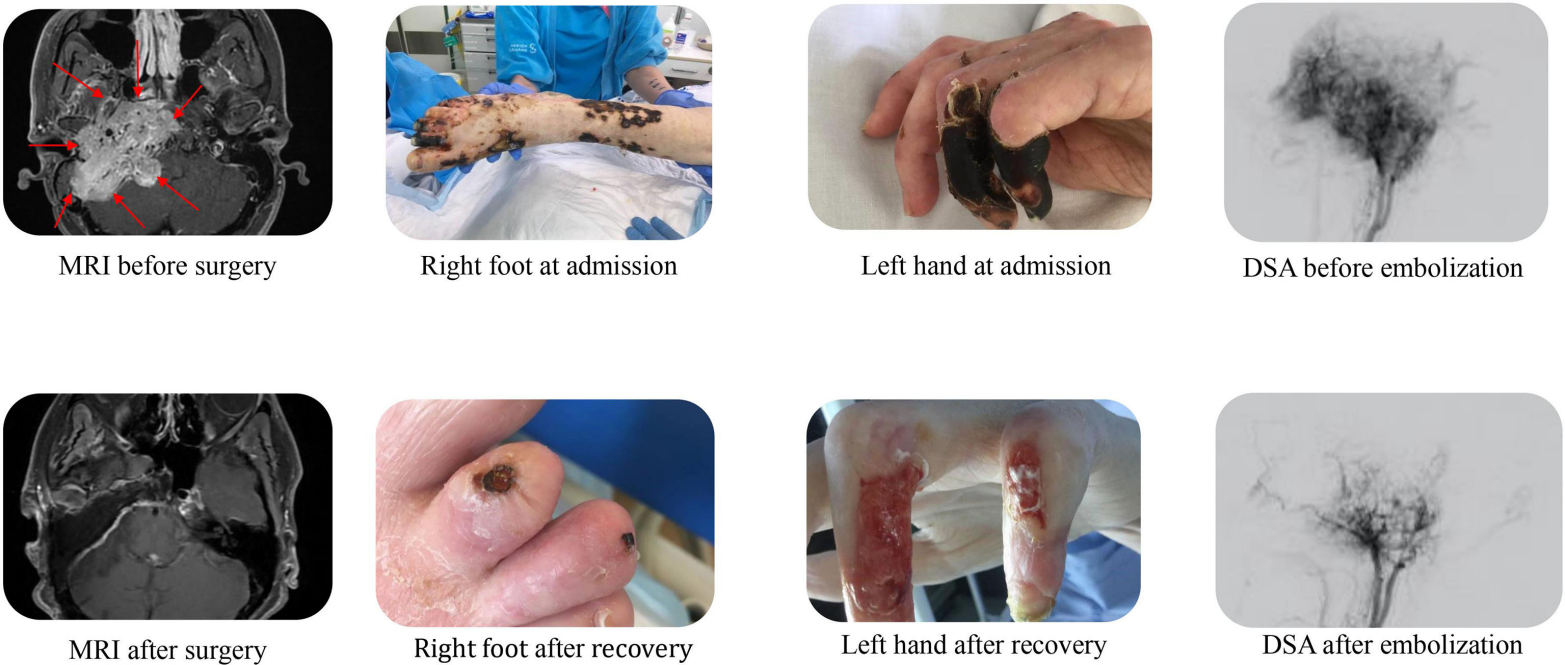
Before and after; MRI scans, pictures of scores, and DSAs. MRI (magnetic resonance imaging) scans before and after tumor resection, clinical pictures of sores on right foot and left hand at admission and at end of hospitalization, and DSA (Digital Subtraction Angiography) images before and after embolization.
